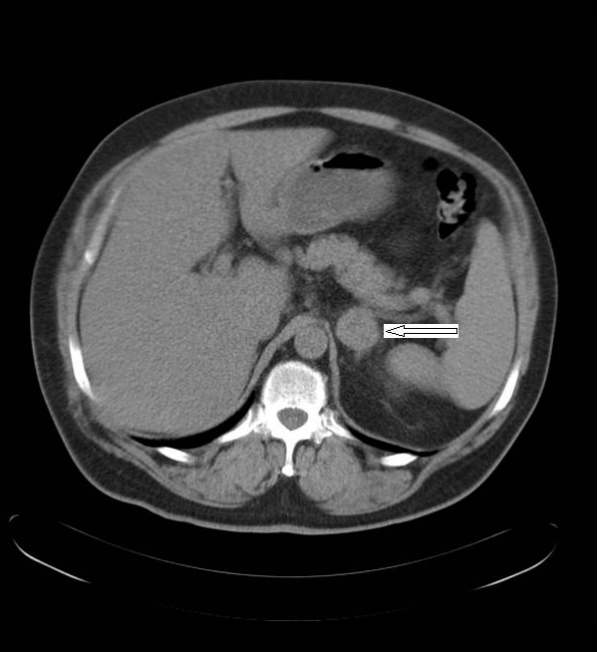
CT scan showing an adrenal metastasis to the contralateral gland, 2 years after a right nephrectomy for primary RCC.
